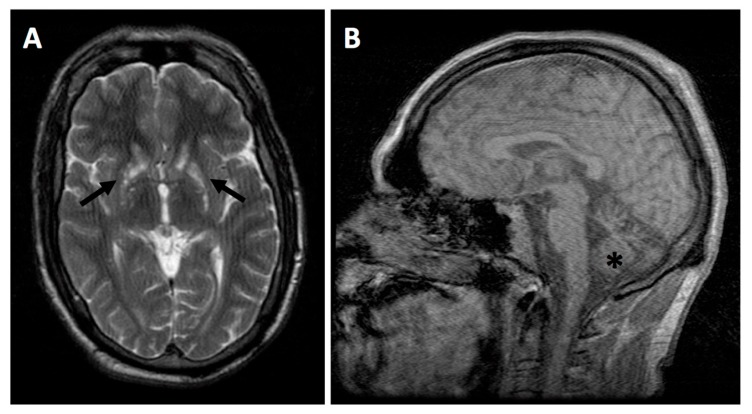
Brain MRI. (A) Axial image demonstrates bilateral, symmetrical T2-weighted high signal intensities within the corpus striatum (arrows). (B) Sagittal image shows cerebellar atrophy (asterisk).
